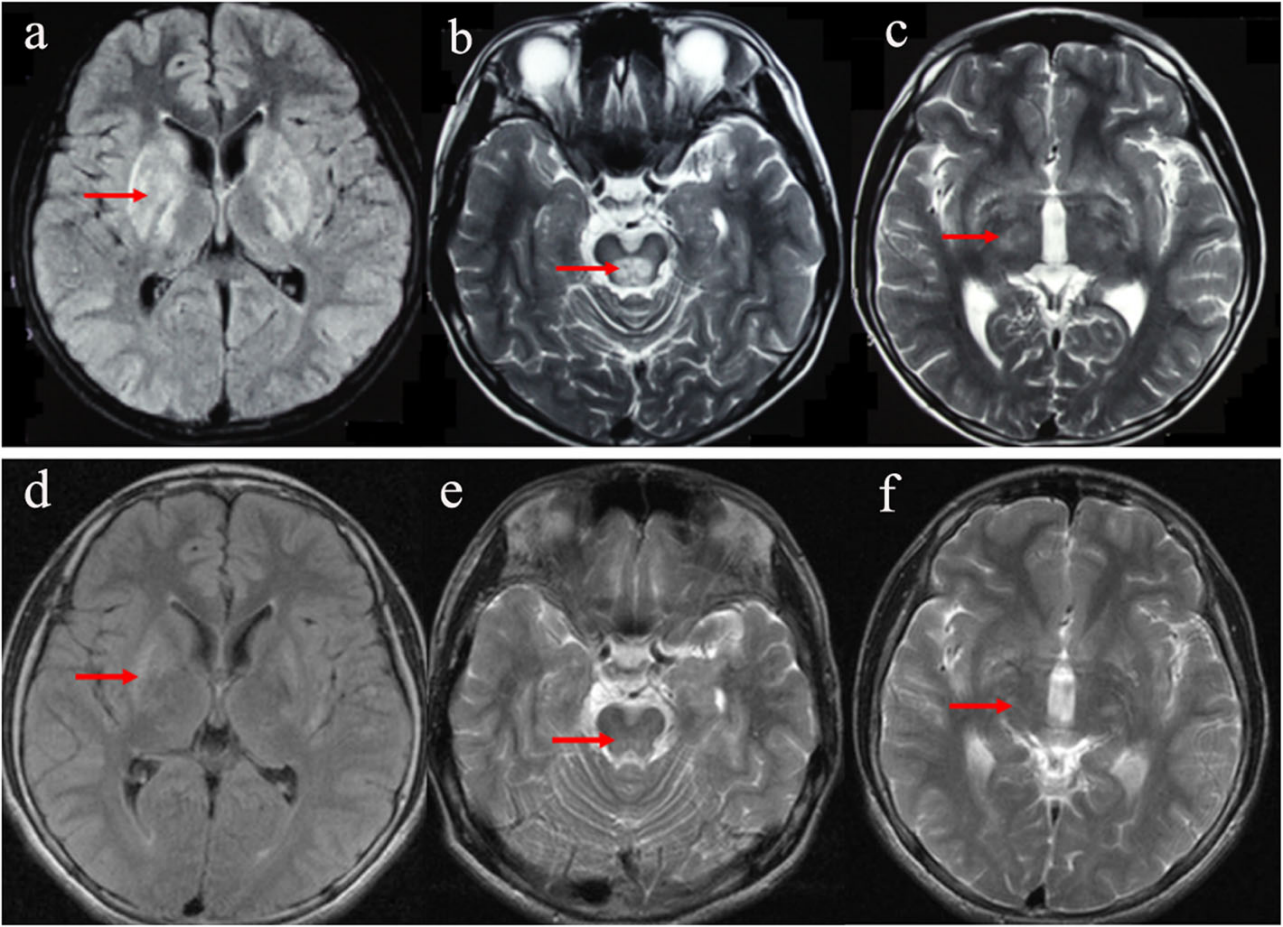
Brain magnetic resonance imaging (MRI) changes in a patient with Wilson’s disease prior to and after 1 year of treatment. Compared with prior treatment (a − c), abnormal signal intensities in basal ganglia, thalamus, and midbrain were all obviously altered after 1 year of combined therapy (d − f)
